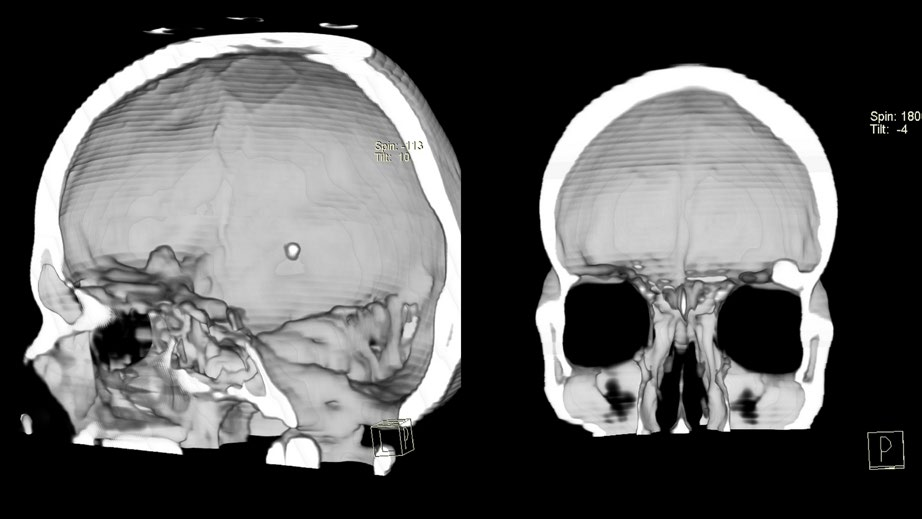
3D CT reconstruction of the skull depicting the mass located in the right anterior cranial fossa
